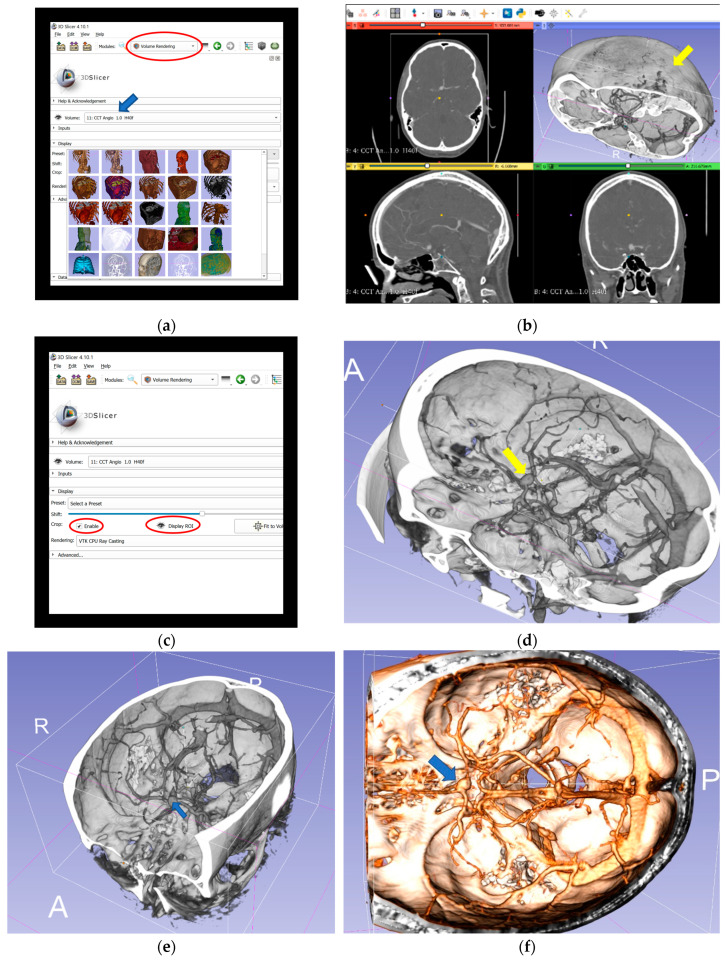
Reconstruction process of 3D-VR images and completion of the final VR scene in 3D Slicer. (a) Import of the original CTA data in anonymized DICOM format into 3D Slicer software to create a patient-specific database and selection of “CCT Angio-Default” (blue arrow) in volume rendering window (red circled). (b) Performance of 3D-VR reconstruction of skull and vessels (yellow arrow) in volume rendering window. (c) Activation of ROI function (red circled), which enables visualization of the aneurysm and relevant vascular anatomy through skull bones from different perspectives. (d) Lateral aspect of the aneurysm (yellow arrow) and relevant vascular anatomy, simplified using ROI function. (e) Oblique lateral aspect of the aneurysm (blue arrow). (f) Superior aspect of the aneurysm (blue arrow) with different color display. 3D, three-dimensional; CCT, cranial computed tomography; CTA, computed tomography angiography; DICOM, digital imaging and communications in medicine; ROI, regions of interest; VR, virtual reality.
